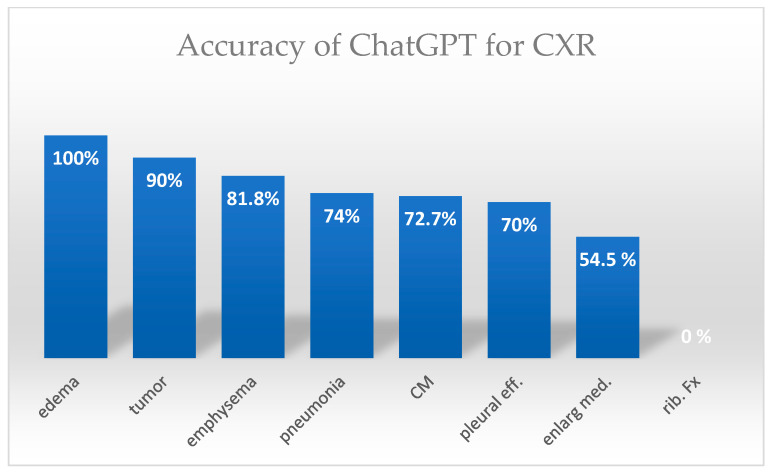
Accuracy of ChatGPT in diagnosing different chest X-ray (CXR) pathologies. CXR diagnoses showed high detection rates for pulmonary edema, tumor, emphysema, pneumonia, cardiomegaly, and pleural effusion, but lower accuracy for pneumothorax, enlarged mediastinum, and rib fractures. Abbreviations: CM = cardiomegaly. Med. = mediastinum. Fx = fracture. Eff = effusion.
